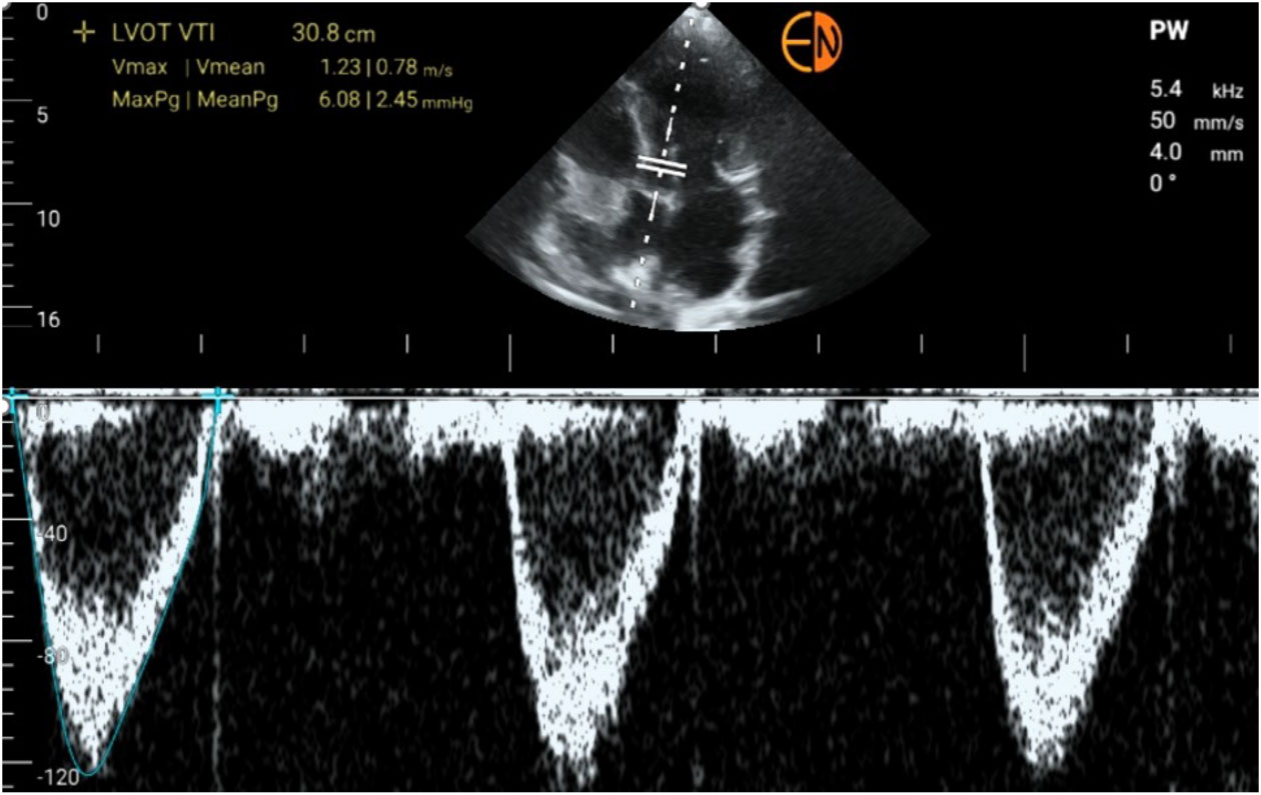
Morphological and functional assessment of the heart using the FoCUS technique. Apical 5-chamber pulsed Doppler view of the LVOT. Note the VTI measurement of 30.8 cm (normal range 17–22 cm). This finding in the setting of acute kidney injury in a patient with cirrhosis can reasonably rule out true hypovolaemia.
